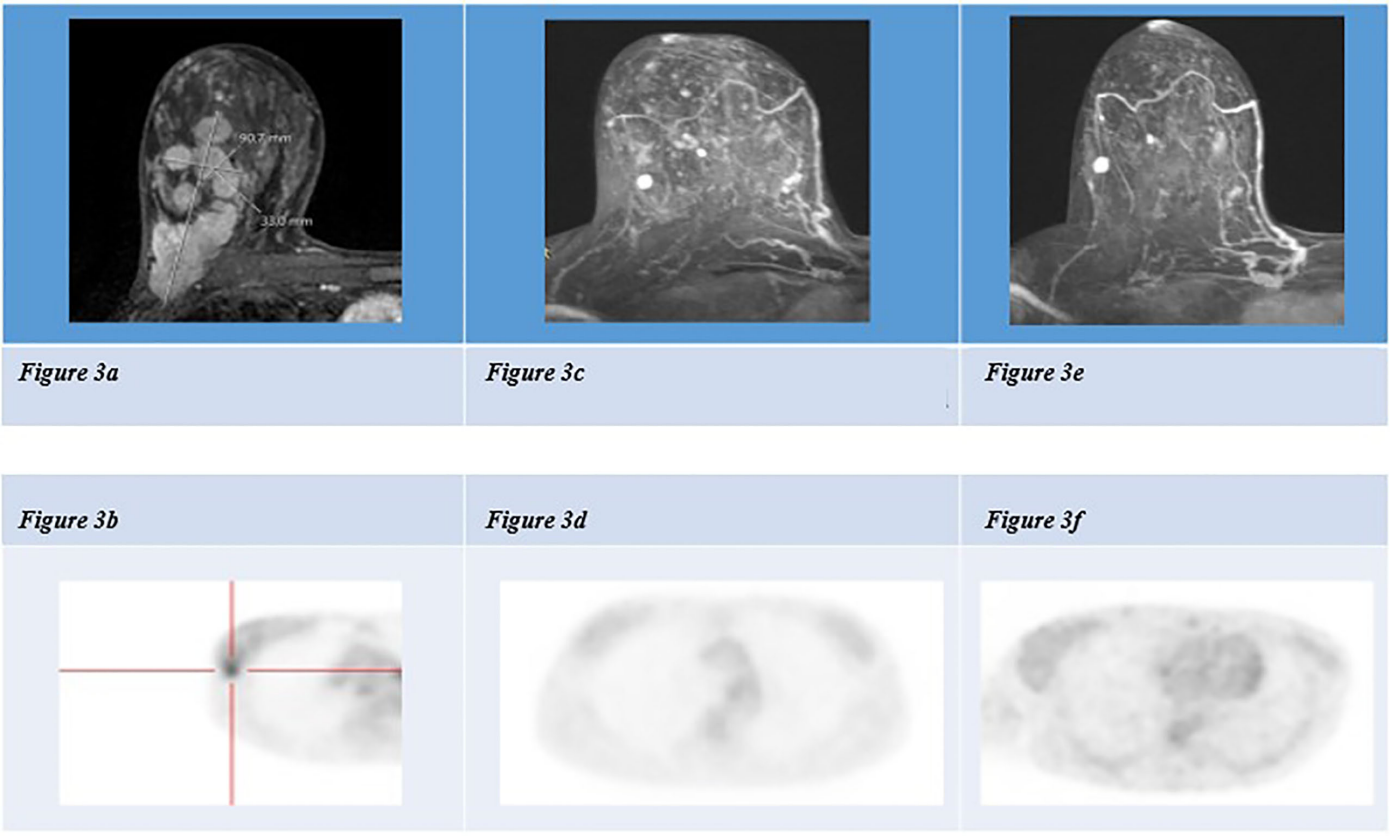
(A) Breast MRI of September 2021: multiple lesions occupying an area of 90x33x60mm in the right breast, below, 18-FDG PET scan of September 2021 showing contrast enhancement in right breast (B). (C) Breast MRI of January 2022: subcutaneous nodules at infero-external and infero-internal right quadrants, with no 18- FDG uptake (see below, D). (E) Breast MRI of May 2022: subcutaneous nodules at infero- external and infero-internal right quadrants, with no 18-FDG uptake (see below, F).
